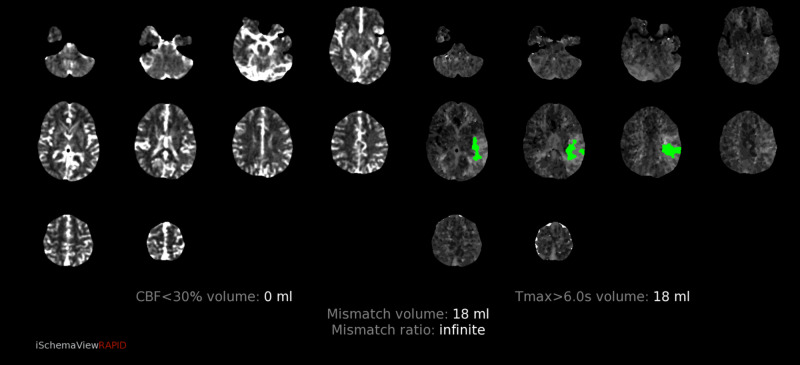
CT perfusion demonstrating mismatch volume of 18mL
